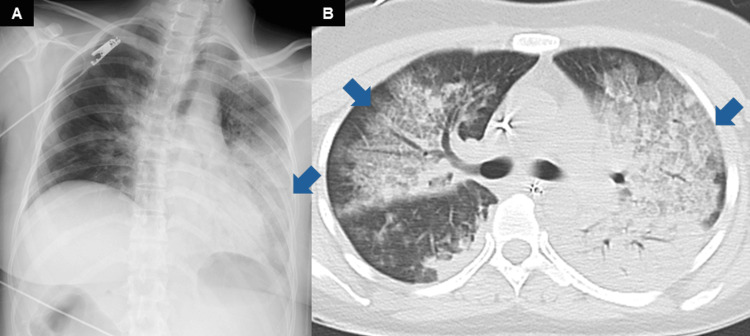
Chest X-ray (A) and computed tomography (B) at emergency department admission
